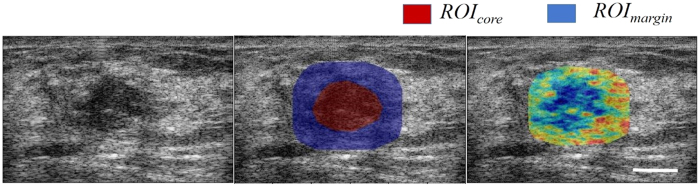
Representative ultrasound B-mode (left), corresponding ROIs with a 5 mm margin thickness (center), corresponding SI parametric image (right). Scale bar: 1 cm.
